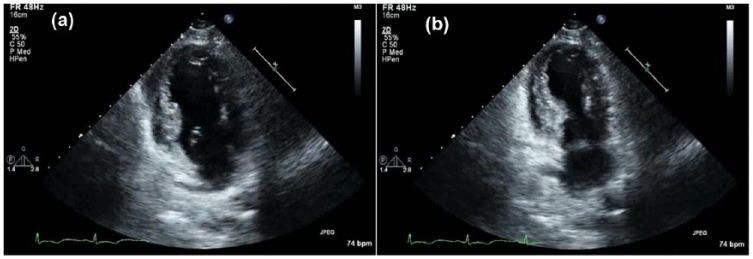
Echocardiogram demonstrating apical hypokinesis: (a) ventricular diastole and (b) ventricular systole.
